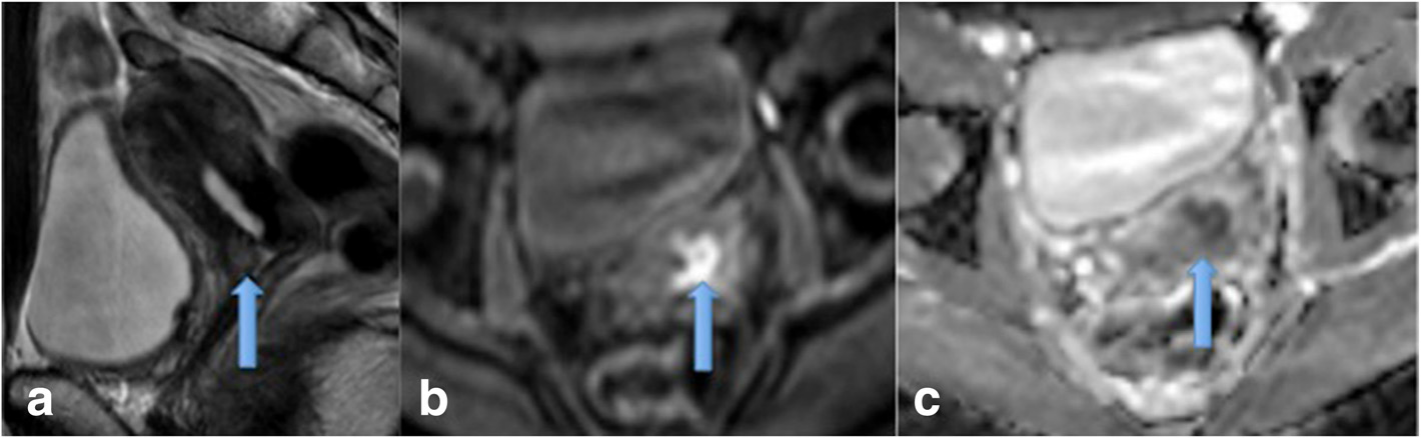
Residual cervical disease. Post treatment images showing a small area of residual disease in the anterior lip of the cervix on sagittal T2 MRI (a) and corresponding restricted diffusion (b and c).
